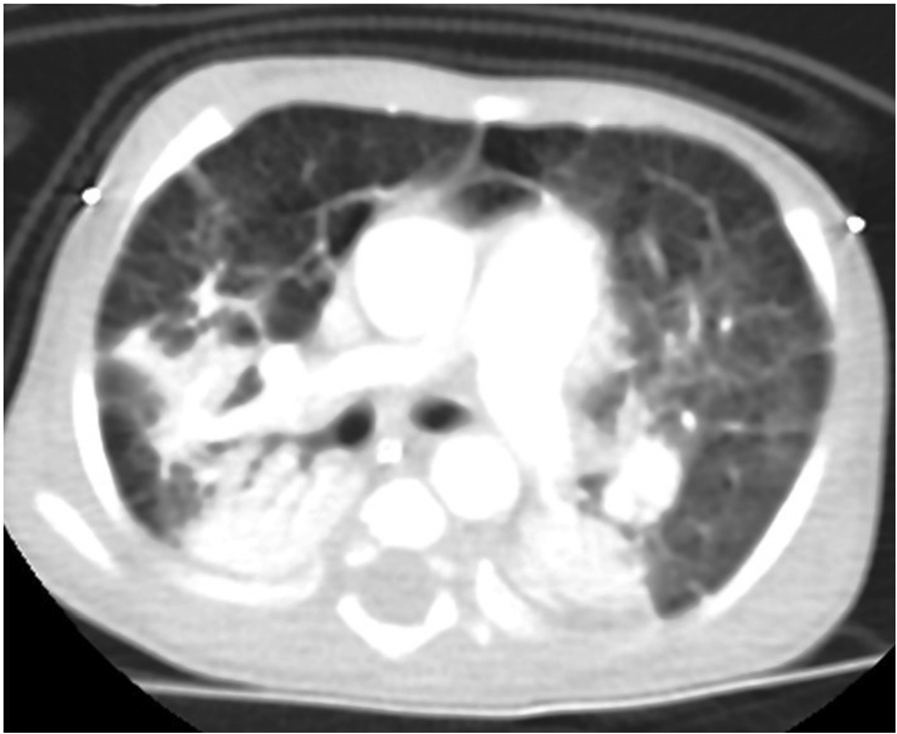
Computed tomography angiography axial view below bifurcation of carina shows basal lung atelectasis, lung hyperinflation, and enlarged main pulmonary artery
